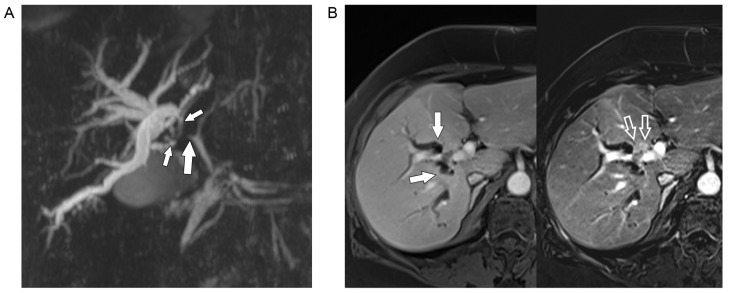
A. MRCP shows stenosis of the right hepatic duct (big solid arrow) extending into the segmental ducts of the right lobe (small solid arrows) with significant biliary dilatation; B. gadolinium-enhanced MRI (left) shows dilated segmental ducts (arrows) converging towards a faintly hyperintense mass anterior to the right portal vein. In the subtracted image (right) the small hypervascular tumor (open arrows) encroaching upon the right portal vein branch is much better seen
